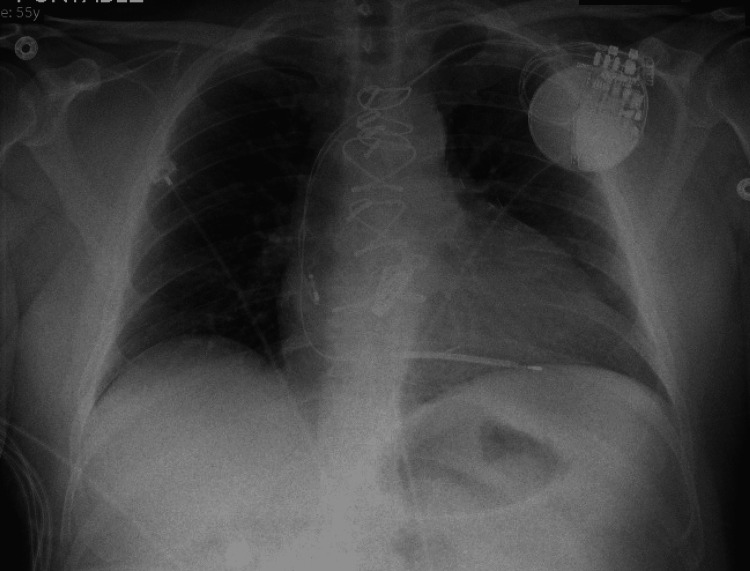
Chest X-ray after the placement of a pacemaker
